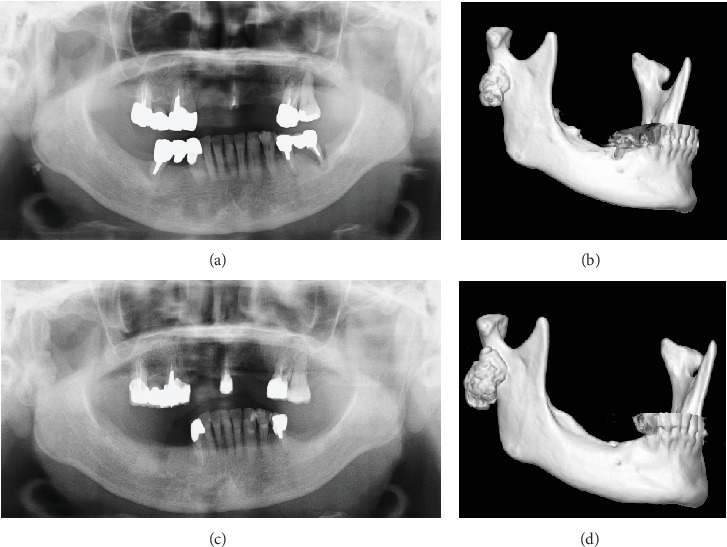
(a, c) Orthopantomography and (b, d) three-dimensional computed tomography images reveal a bony lesion at the right subcondylar region of the mandible. (a, b) The first visit. (c, d) Nine years after the first visit.
